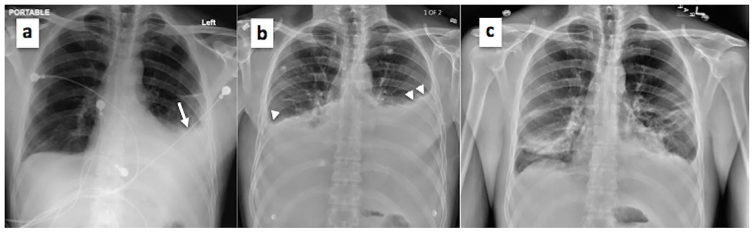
Plain radiograph of the chest showing (a) obliteration of the left costophrenic angle on initial presentation (white arrow); (b) reaccumulation of chylothorax with bilateral obliteration of costophrenic angles on second patient admission (white arrow heads); and (c) resolution of chylothorax effusion prior to final patient discharge.
